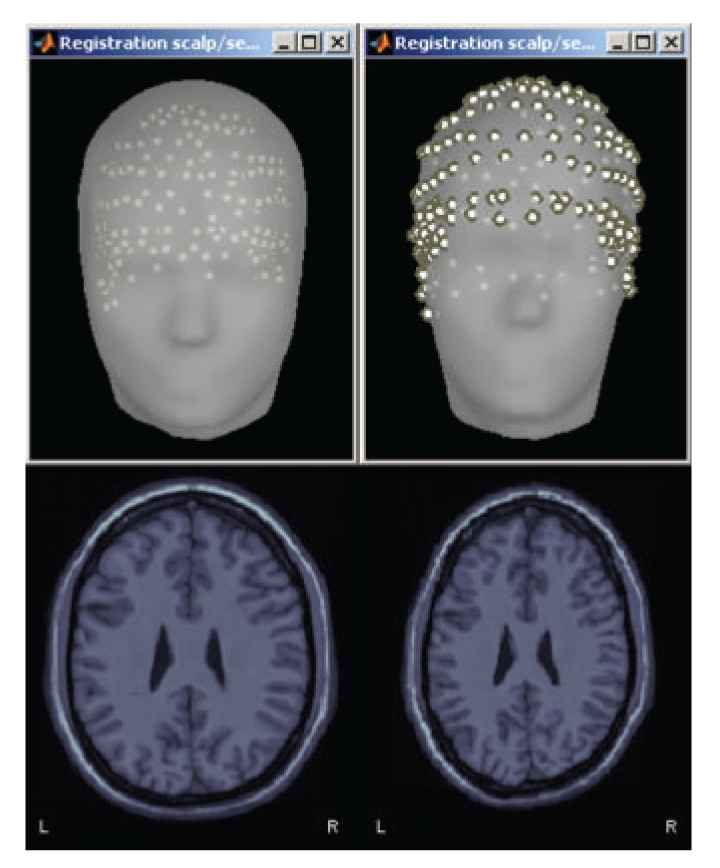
Warping of the MRI volume and corresponding tissue surface envelopes of the Colin27 template brain to fit a set a digitized head points (white dots in upper right corner): initial Colin27 anatomy (left) and warped to the scalp control points of another subject (right). Note how surfaces and MRI volumes are adjusted to the individual data.
